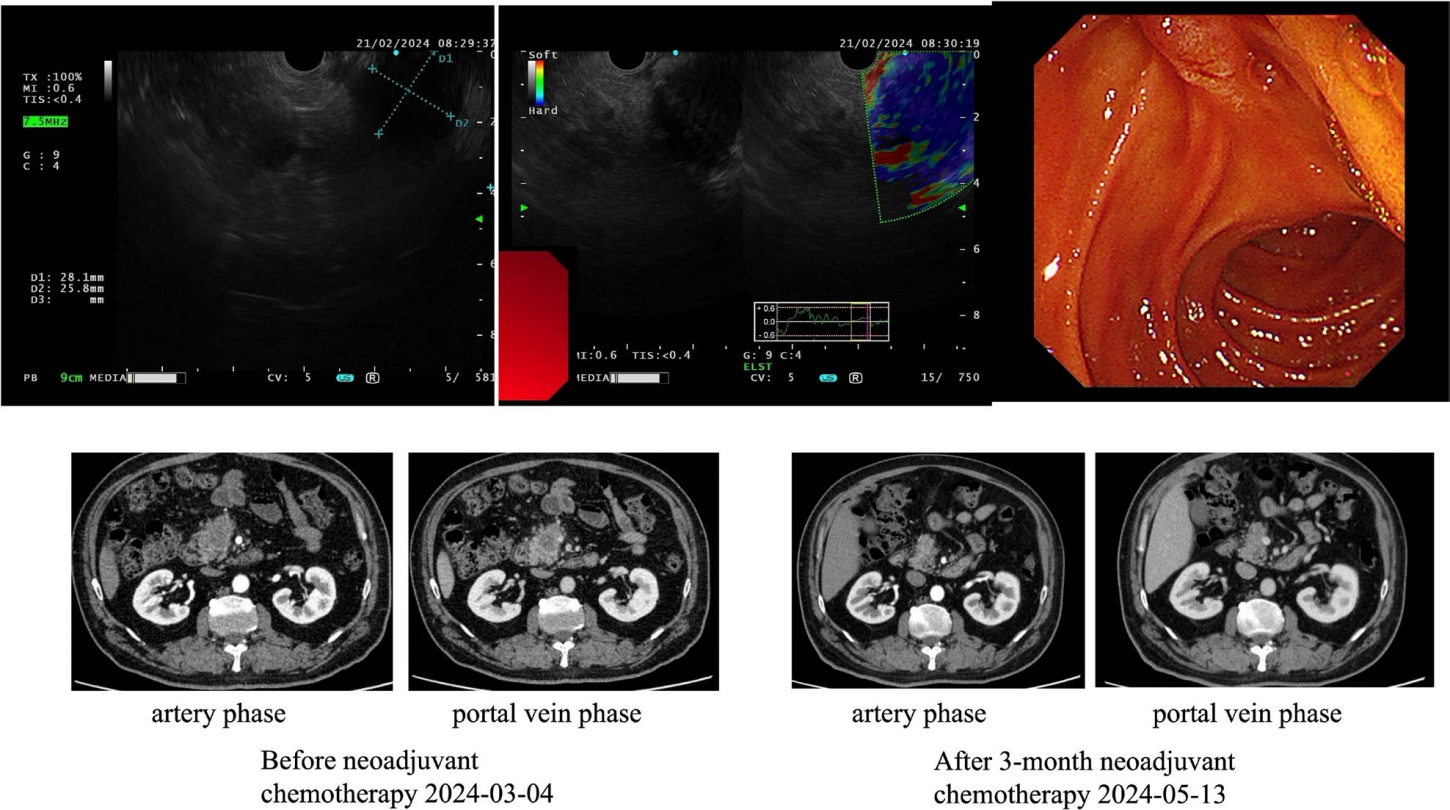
FNB‐EUS results and enhanced CT results. The endoscopic ultrasonography showed a hard mass in the pancreatic head. The enhanced CT scan indicated that after three cycles of chemotherapy, the tumor had significantly shrunk, and the involvement of the superior mesenteric vein (SMV) had decreased compared to the previous state.
